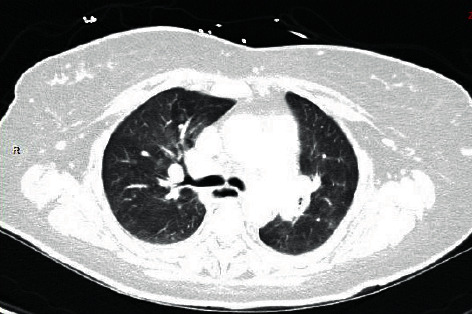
Chest computed tomography revealing mediastinal and hilar lymphadenopathy.
